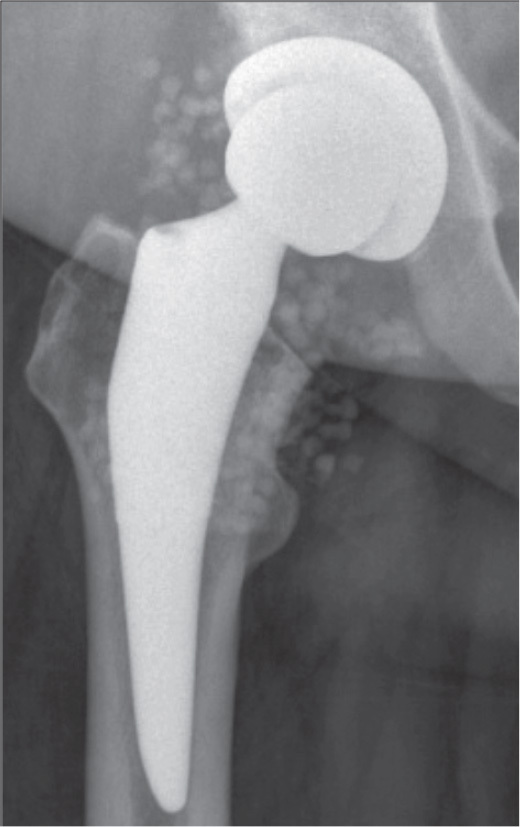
Anteroposterior radiograph after a DAIR procedure with application of calcium sulphate beads CS of 20 mL antibiotic-loaded rapid cure powder mixed with 2 g vancomycin and 480 mg gentamicin for an early postoperative periprosthetic hip infection with *Enterobacter cloacae* in a 57-year-old female patient.
